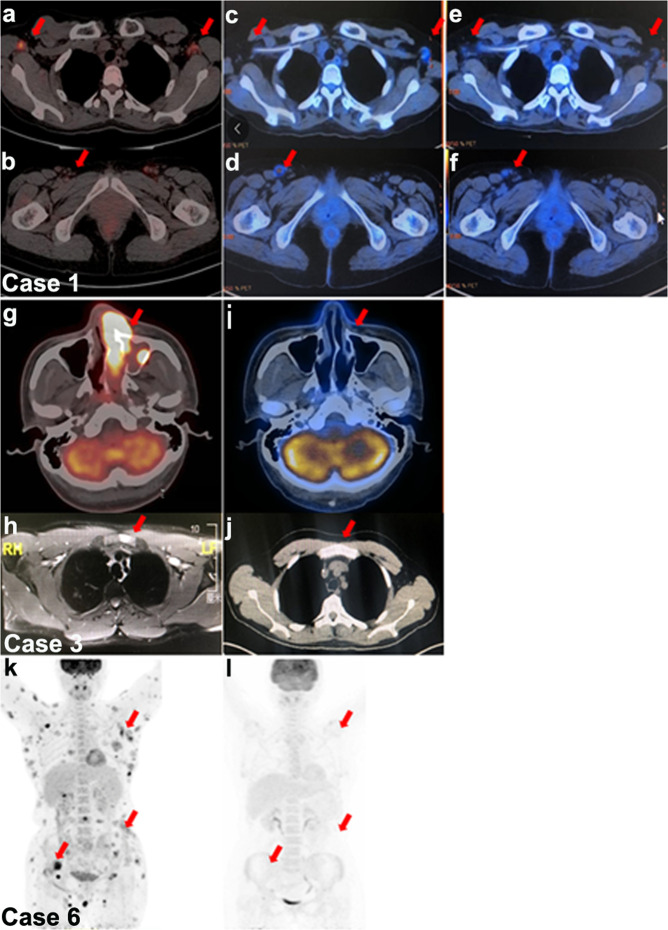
Imaging results of three representative patients. a–f PET/CT results of case 1. Baseline PET/CT showed multiple lymph nodes involvement (a, b). After the sixth cycle of conventional chemotherapy, there were persistent FDG-avid lesions by PET/CT scan (c, d). After 2 cycles of immunochemotherapy, no FDG-avid lesions were observed and CR was confirmed (e, f). g–j PET/CT and MRI results of case 3. Baseline PET/CT and MRI examination showed neoplastic metabolic lesions invading the nasal cavities and sinuses, with MRI-proved lymphomatous infiltration in the sternal manubrium (g, h). After 4 cycles of immunochemotherapy, no FDG-avid lesions were found in a follow-up PET/CT scan, and the manubrium sternum lesions completely disappeared; demonstrating CR (i, j). k, l PET/CT results of case 6. Baseline PET/CT scan of case 6, presenting no nasal disease but systemic tumor invasion of the skin and lymph nodes (k). After 6 cycles of immunochemotherapy, all original skin lesions resolved, and no FDG-avid lesions were seen in the PET/CT scan, which indicated a response of CR (l)
